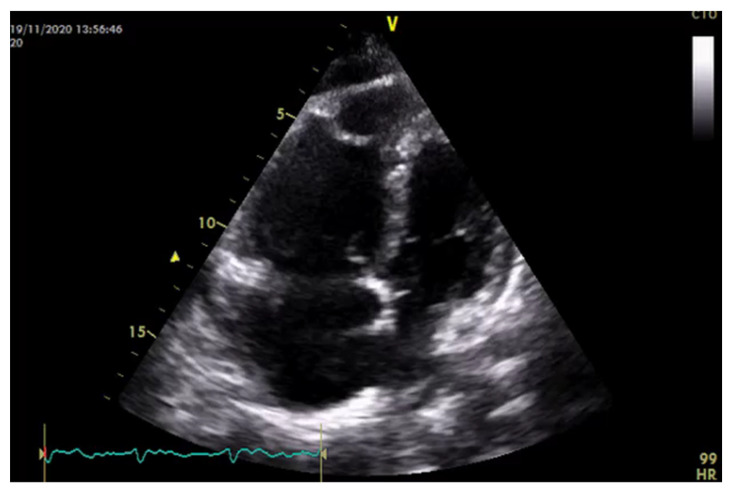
A 4-chamber view of standard echocardiography in a patient with PH. As results of the increased afterload, RV wall hypertrophies and dilates, developing a spherical shape accompanied by increased RV wall stress, RV dysfunction and severe RA dilatation.
